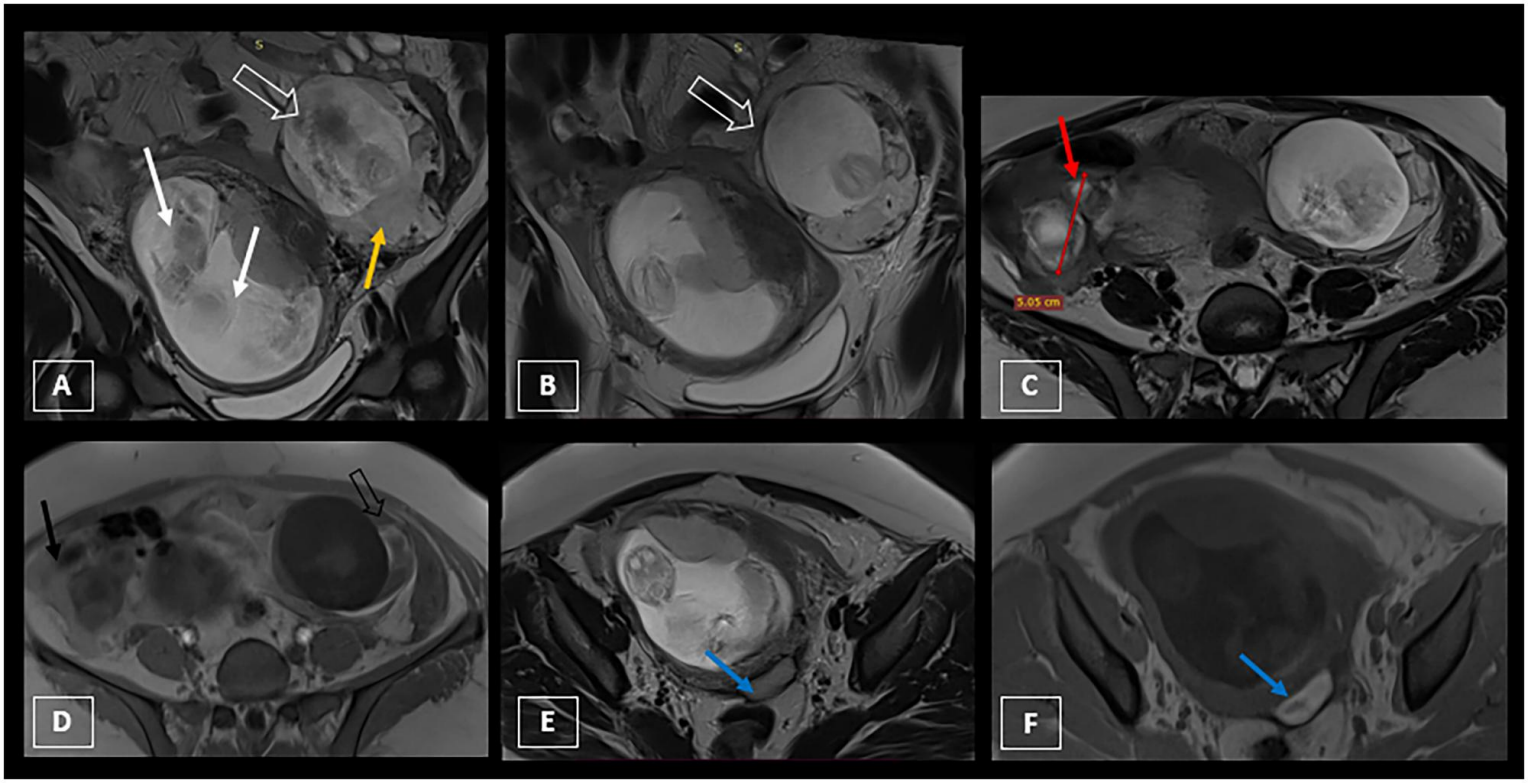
Pelvic MRI showing a heterotopic triplet pregnancy. (A and B) Coronal T2-weighted images showing a bifoetal, bichorionic, and bi amniotic intrauterine pregnancy (white arrows) associated with a third gestational sac lateralized to the left, located intra-abdominally (empty white arrow), not surrounded by myometrium, with its own placenta (yellow arrow). (C) T2-weighted axial image showing the free ectopic sac in the abdominal cavity. Note the right ovary that is enlarged and multi-follicular (red arrow), consistent with ovarian hyperstimulation syndrome. (D) T1-weighted axial section showing blood around the ectopic placenta (black empty arrow) and haemoperitoneum (black arrow), suggesting signs of early rupture of the ectopic gestational sac. (E) T2-weighted axial section and (F) T1-weighted axial section showing an associated left haematosalpinx (blue arrow) that is probably due to an undetected rupture of the fallopian tube, which resulted in the extrusion of the zygote into the peritoneal cavity, suggesting a secondary origin of the abdominal heterotopic pregnancy.
